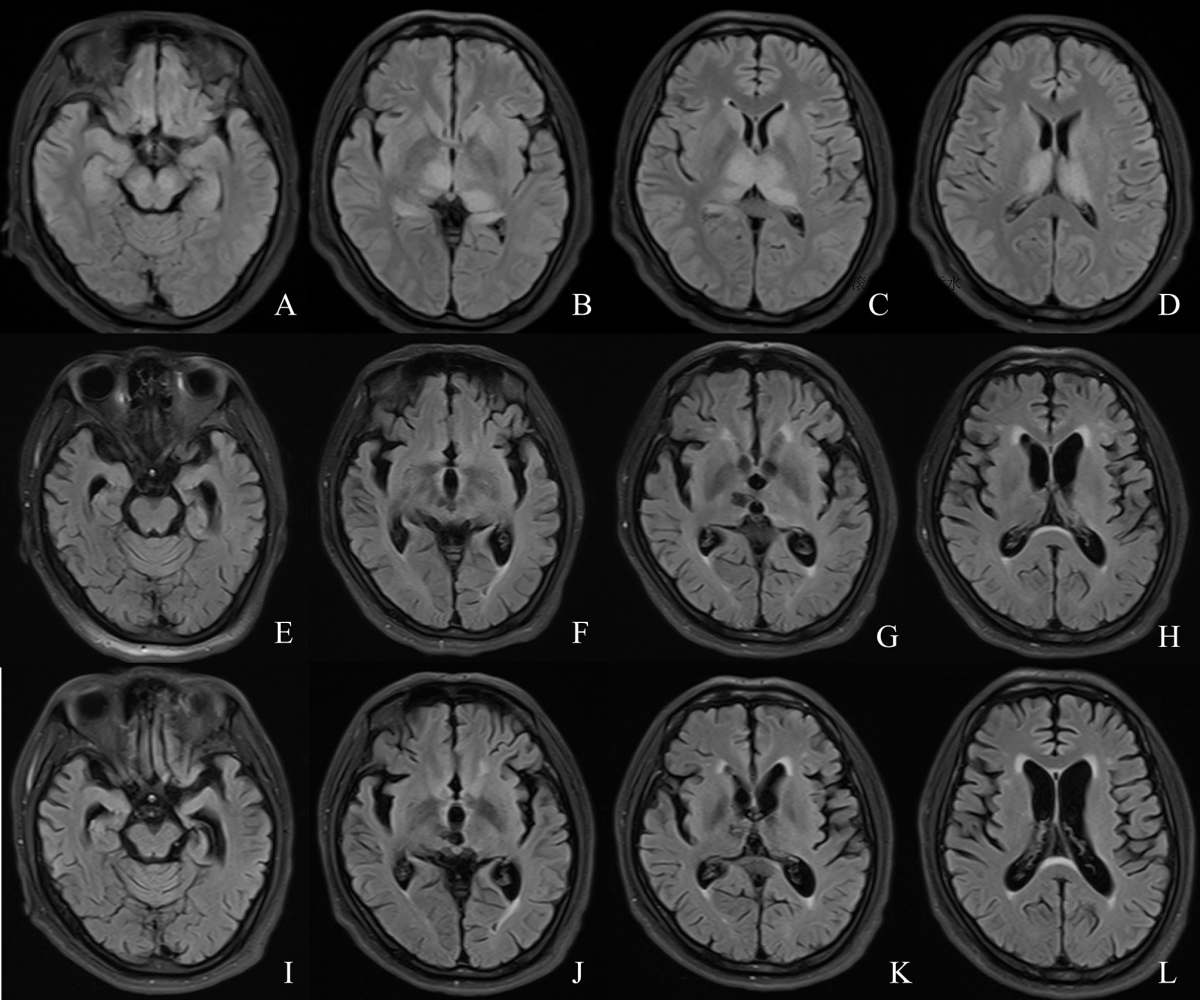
Comparison of the patient’s MRI. (A–D) MRI on day 6 of onset. (E–H) MRI re-examination at month 6 of the course of disease. (I–L) MRI examination at month 14 of the course of disease.
